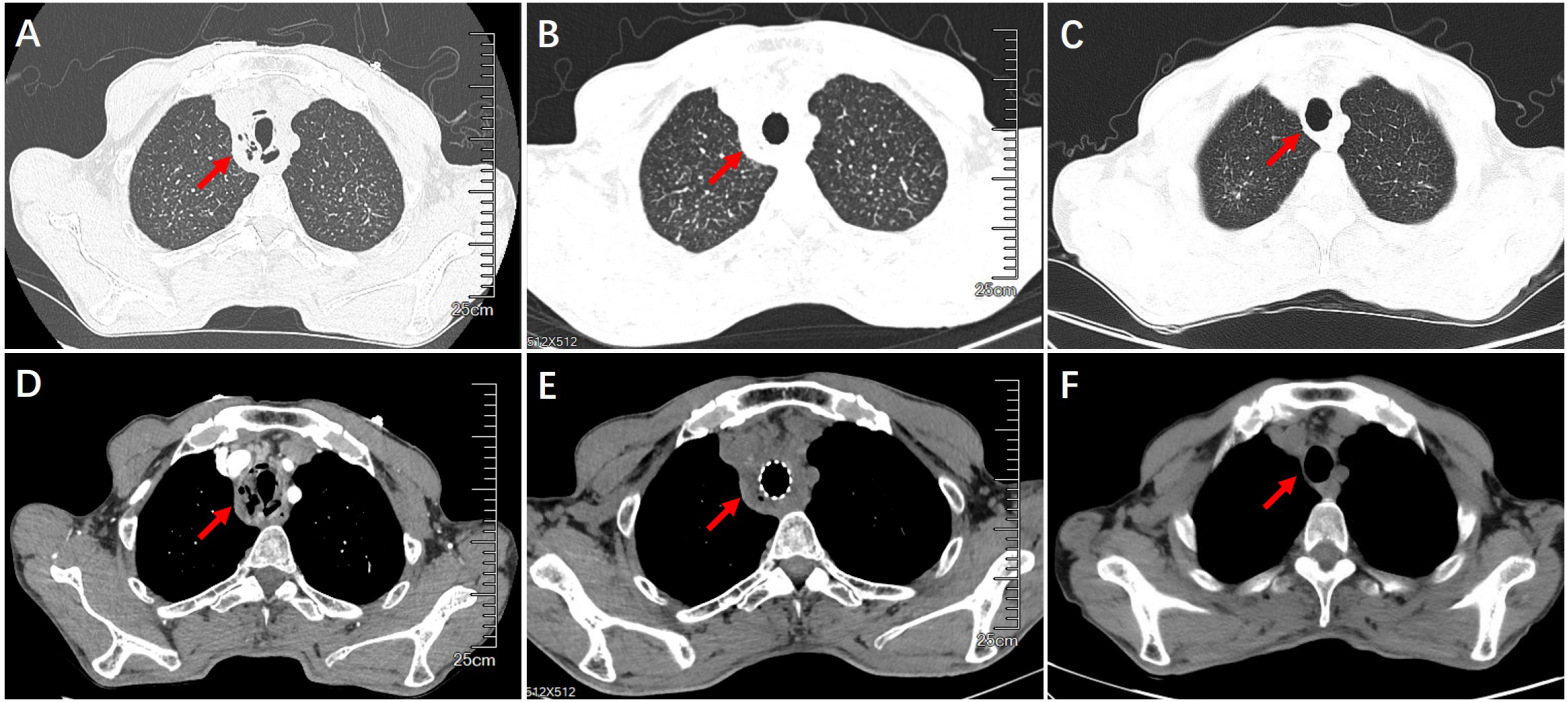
Chest CT imaging on airway structural changes before and after treatment. (A, D) Airway wall thickening, necrosis and luminal obstruction before treatment. (B, E) Airway patency after the placement of airway stent. (C, F) Improvement of tracheal wall thickening and luminal narrowing after interventional treatment with airway stent withdraw.
